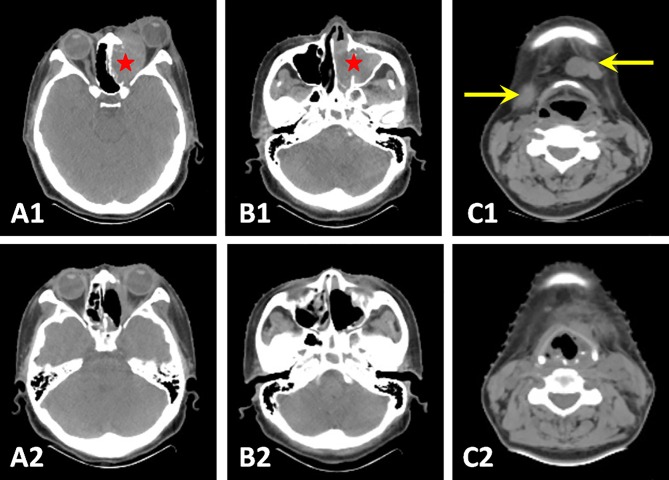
Pre- and post-operative CT scans. Endoscopic surgery released intraorbital compression behind the left eyeball (A1 and A2, red star) and resected tumor mass in left nasal cavity, maxillary and ethmoidal sinus (A2, B1and B2). Elective neck dissection removed those metastatic cervical lymph nodes (C1 and C2, yellow arrows). Those post-operative CT scans were taken after 2 months of surgery.
